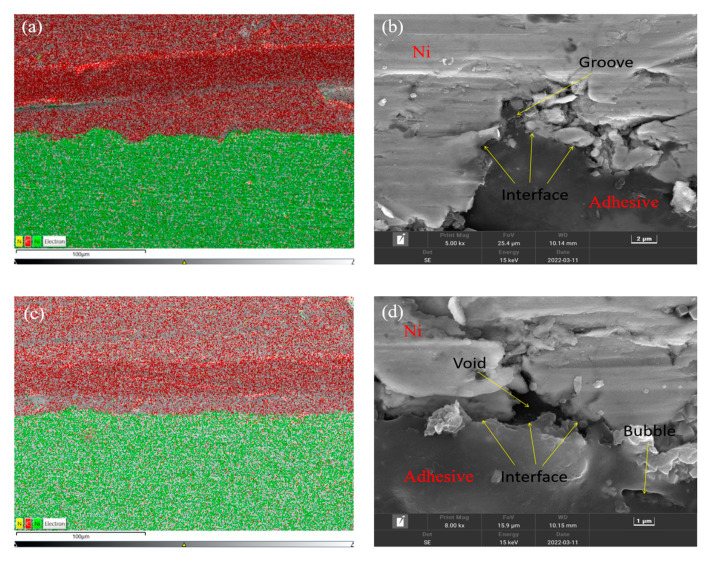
EDS mapping images of the interface (a) with and (c) without the ultrasonic treatment, and SEM images of the interface (b) with and (d) without the ultrasonic treatment.
